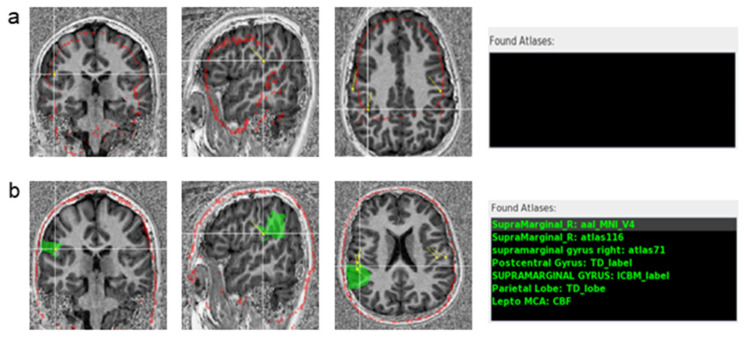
Skull registration validation on an MP2RAGE T1-weighted MRI. (a) Registration using FLIRT: the skull model (red outline) does not match the patient’s MRI. (b) Registration in the same subject using CAT12: the skull model (red outline) matches the skull on the patient’s MRI. The atlases’ labels (in green) for the selected dipole are displayed on the right of the image (Found Atlases). On the top, using FLIRT registration, which failed in this patient, no atlas was found. On the bottom, the Found Atlases section lists the different brain atlases and the corresponding labels (green) for the crosshair dipole, using CAT12. The selected dipole is correctly labeled by the AAL atlas as the right supramarginal gyrus, highlighted by the green overlay. In Found Atlases, the abbreviation is as follows: R, right.
